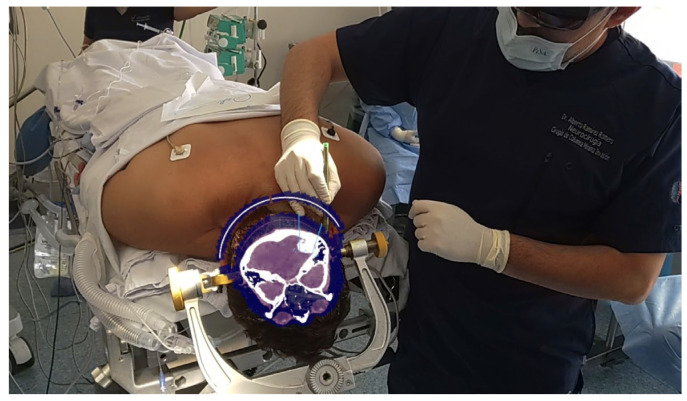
Target placement with AR, where the patient’s CT scan can be observed in the coronal view.
